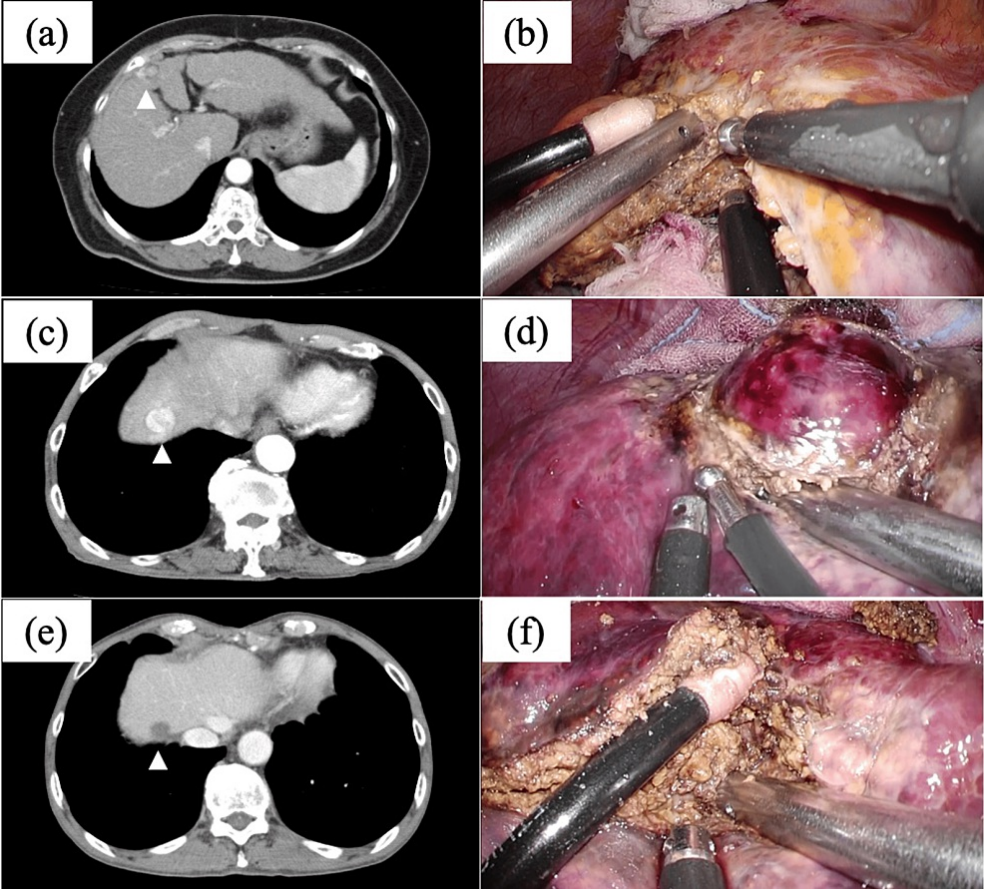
CT images and intraoperative findings of cases 2, 4, and 5 (a, b) In case 2, a 2.5-cm-sized tumor in S4/S5 was detected (arrowhead), and non-anatomical liver resection was performed utilizing WJS. (c, d) In case 4, a 1.5-cm-sized tumor in S8 was well-enhanced in an arterial phase (arrowhead), and S8 non-anatomical liver resection was performed using WJS. (e, f) In case 5, a well-demarcated 2.0-cm-sized tumor was detected in S5/S8, and non-anatomical liver resection of S5/S8 was performed in the same manner. CT; computed tomography, WJS; water jet scalpel, S4; segment 4, S5; segment 5, S8; segment 8
